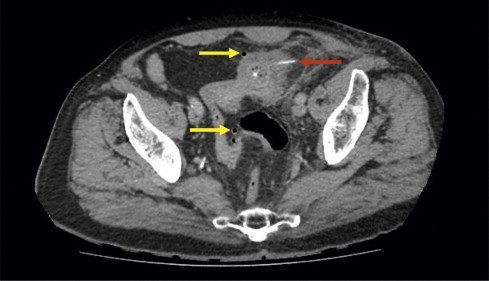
CT scan of abdomen and pelvis showing radio-dense bony foreign body perforating through the sigmoid colon (red arrow). Flecks of free intra-peritoneal air can be seen (yellow arrows).
